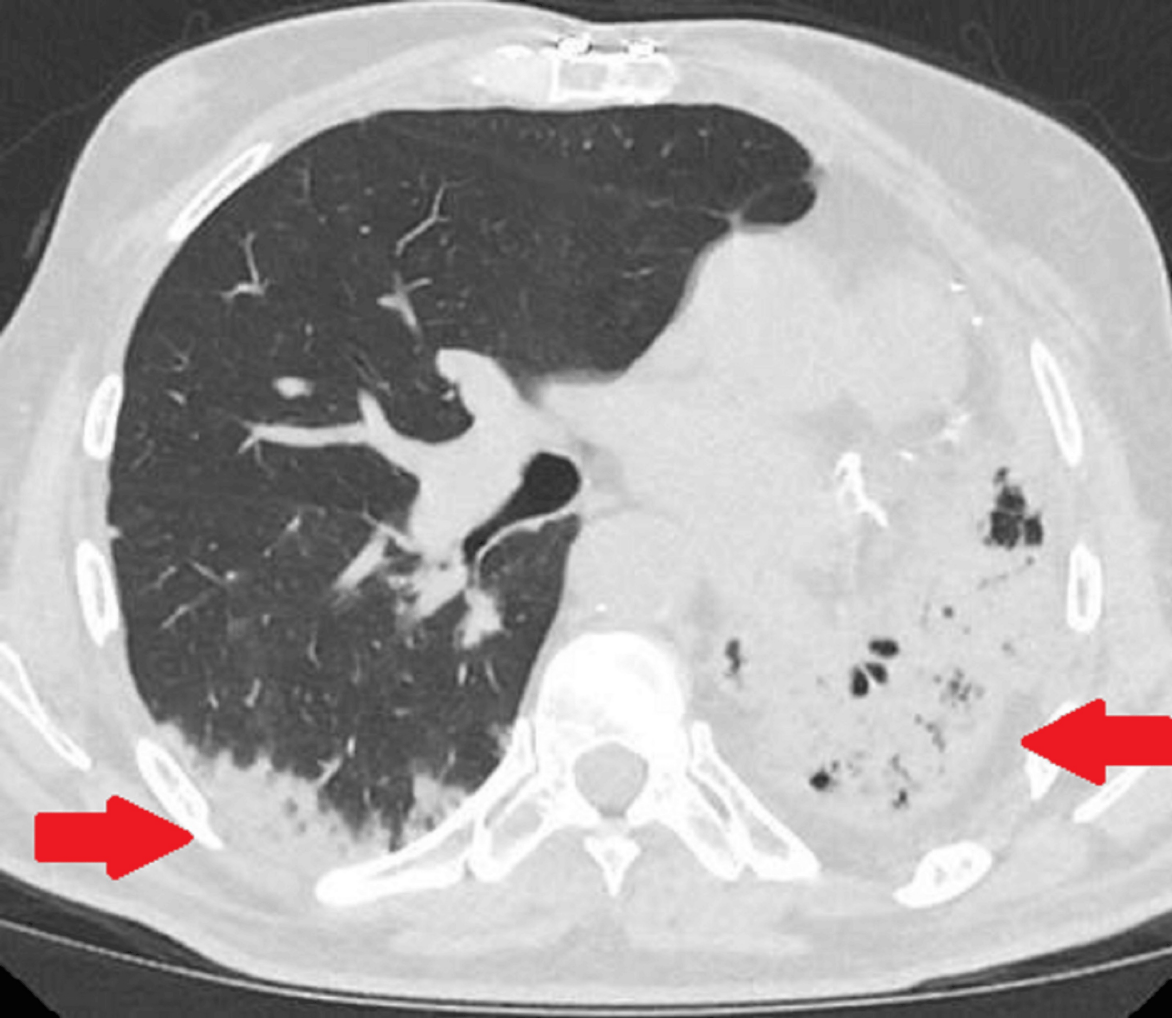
Follow-up axial non-contrast-enhanced CT lung-window image. The image obtained three months later demonstrates progressive ground-glass and solid opacities in the lower lobes, more pronounced on the left than the right, with a background of fibrosis in the left lung (red arrows).
